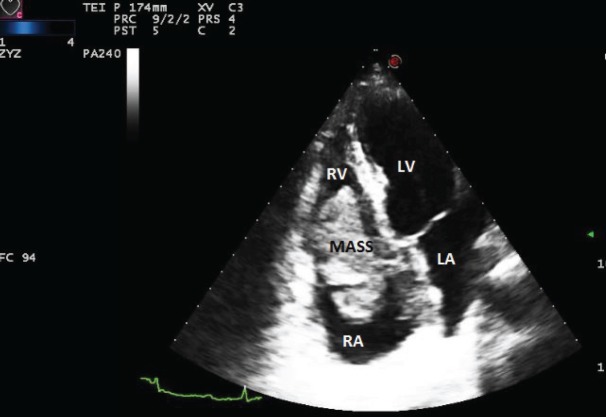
The transthoracic echocardiogram showed a mass located between the right atrium and the right ventricle. LV: left ventricle, LA: left atrium, RV: right ventricle, and RA: right atrium.
